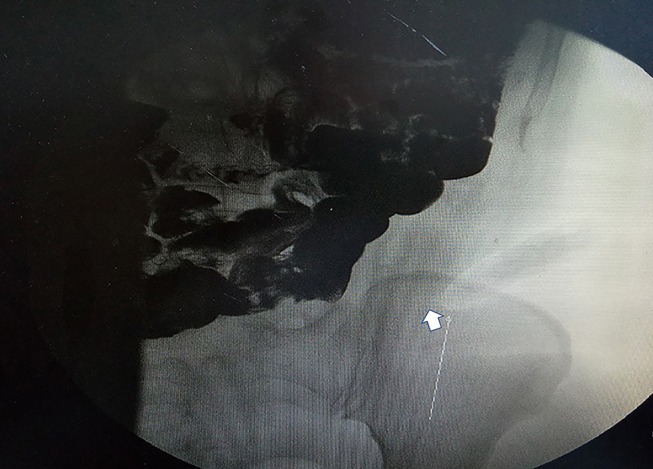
Gastrointestinal radiography revealing a mass in the left lower quadrant. Arrow points to an oval tumor (white arrow).
